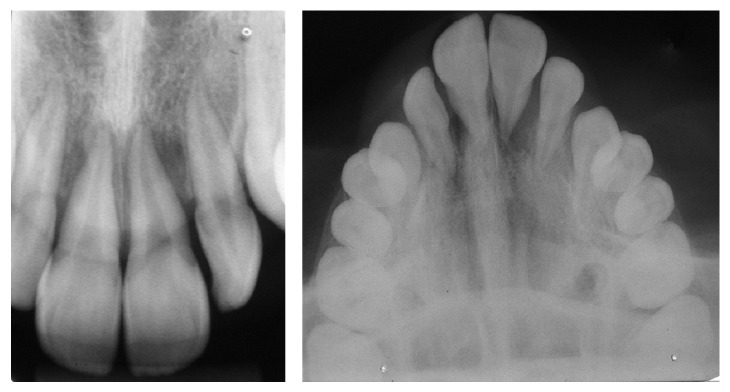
Initial radiographic findings.
